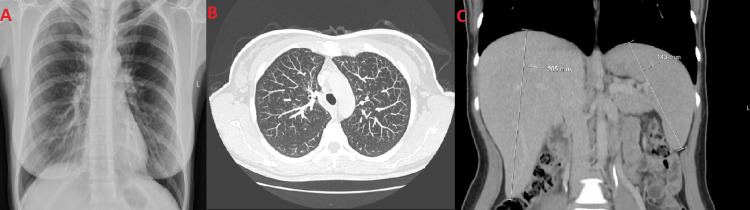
A: Chest x-ray was unaltered; B: Axial CT section of the chest; C: Coronal CT Abdomen showing hepatomegaly of 205 mm and splenomegaly of 143 mm
